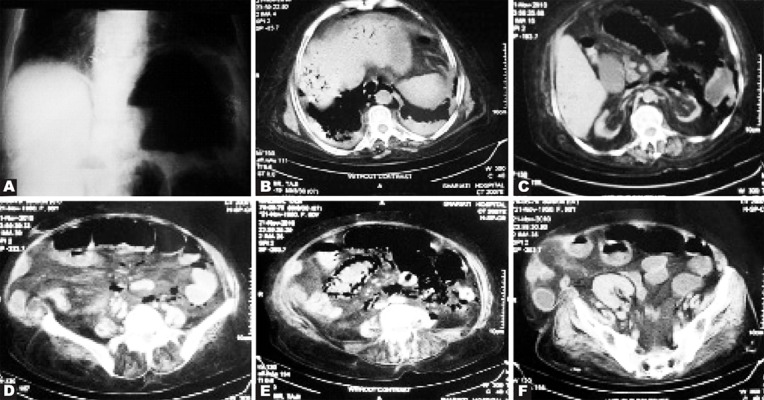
A 59-year-old woman with nausea, vomiting and generalized abdominal pain after colonoscopy. A. Plain abdominal graphy shows dilation of bowel loops and air in the subdiaphragmatic and retroperitoneal area. B-F. Contrast enhanced abdominopelvic CT scan shows free air in the retroperitoneal, intraperitoneal and posterior mediastinal spaces with pneumatosis intestinalis and gas bubble in the portal vein branches. Note the transplant kidney and atrophic native kidneys and right abdominal wall incisional hernia. Bilateral pleural effusion and ascites is evident too.
